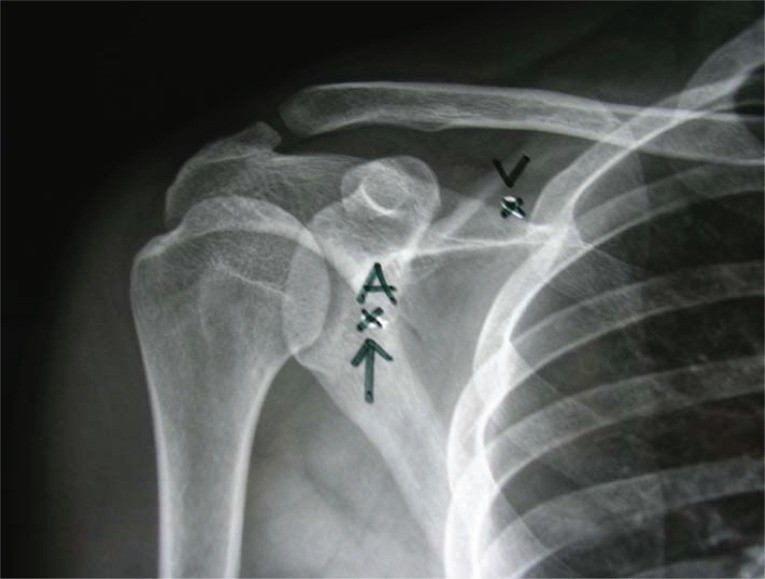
Posterior-anterior X-ray shows the distance between the neddle insertion point and the thorax wall.
